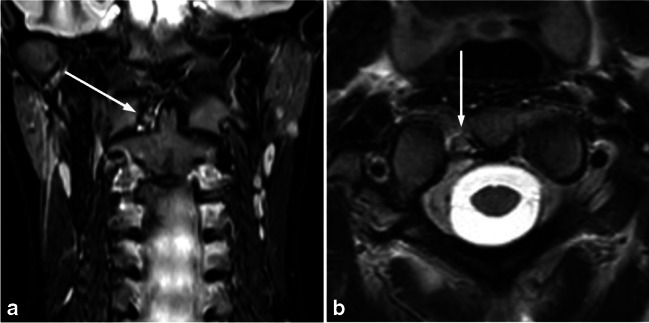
Partial tear of the right transverse ligament (*arrows*) in a 14-year-old girl after a basketball accident. a Coronal short tau inversion recovery magnetic resonance image (MRI). b Axial T2-weighted MRI
